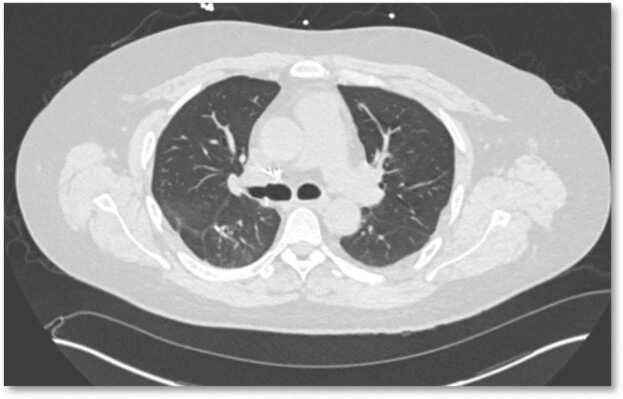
Follow-up chest CT axial image with demonstration of resolution of the bilateral ground glass opacities.
